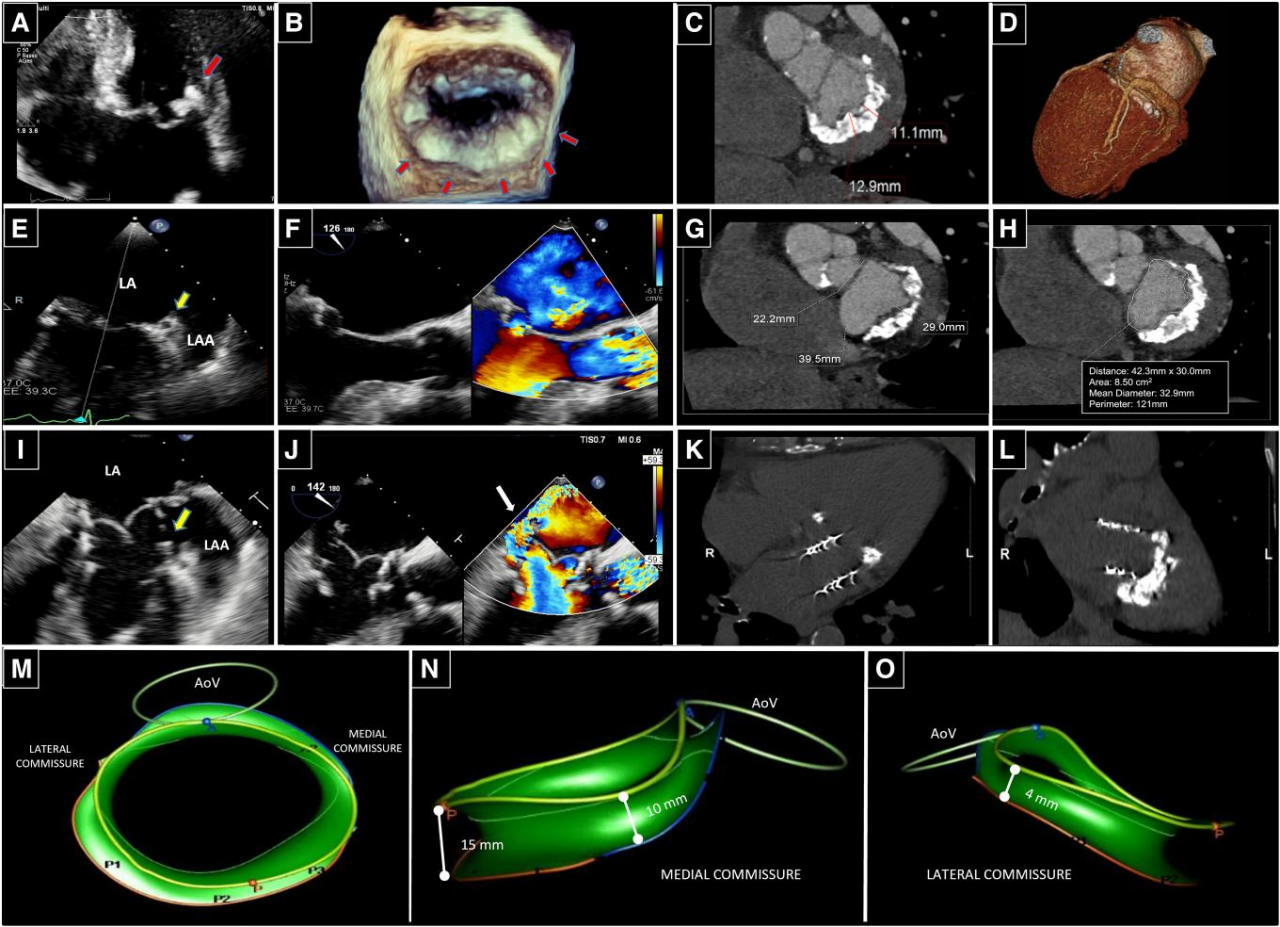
Multimodality imaging of MAC before (*A–H*) and after (*I–L*) surgery. Pre-operative transthoracic (*A*) and 3D transoesophageal echocardiography (TEE) *en face* (*B*) views showing an extensive MAC of the posterior mitral annulus (*arrows*). Pre-operative contrast-enhanced computed tomography (CT) short-axis (*C*, *G*, *H*) MPR images and volume rendering (*D*) showing *radial* calcium thickness > 10 mm, large mitral annulus diameters (29 mm anteroposterior, 39.5 mm bicommissural) and anatomical area (8.50 cm^2^), a nearly normal intertrigonal distance (22.2 mm), and calcium distribution > 270° with incomplete extension in the annular circumference (MAC score 8). TEE bicommissural (*E*, *I*) and long-axis (*F*, *J*) views showing pre-operative MAC (*E*, *F*) and post-operative severe PVL (*J*, *arrow*), extending from the posterior portion of the mitral annulus to the lateral commissure, near the circumflex artery (*I*, *arrow*). Long-axis (*K*, *L*) MPR images from the post-TMVR unenhanced CT showing device position, proximally to the MAC and protruding within the left atrium (LA). *3D-TEE-modified MV model* obtained by starting from the AI-based 3D MV annulus modelling application analysis, implemented into the TomTec software (TomTec-Arena, version TTA2.30.02, Philips, Italy; commercially available, standard across institutions) (*M–O*): by properly selecting planes and positioning reference points along the mitral annulus, at the MV leaflet coaptation, and at the aortic virtual basal ring, the AI-based software automatically provides a 3D MV reconstruction model which clearly shows planar or saddle-shape features, annular dimensions, ellipticity or sphericity, absolute displacement and displacement velocity, mitral-aortic angle, and leaflet extent. Once the automatic reconstruction is obtained, the model can be manually modified by moving the lines, which depict the leaflets, towards the ventricular side of the annular calcium, thus enveloping the entire calcium extent. In experienced hands, it takes about 30–60 min for the manual adjustments. Our model showed a nearly preserved saddle-shaped calcified mitral annulus along with an asymmetric *cranio-caudal* distribution of calcium thickness, greater at the posterior level (15 mm) and less represented at the lateral commissure (4 mm), where the PVL occurred. AoV, aortic valve; LA, left atrium; LAA, left atrial appendage; MPR, multi-planar reconstruction.
